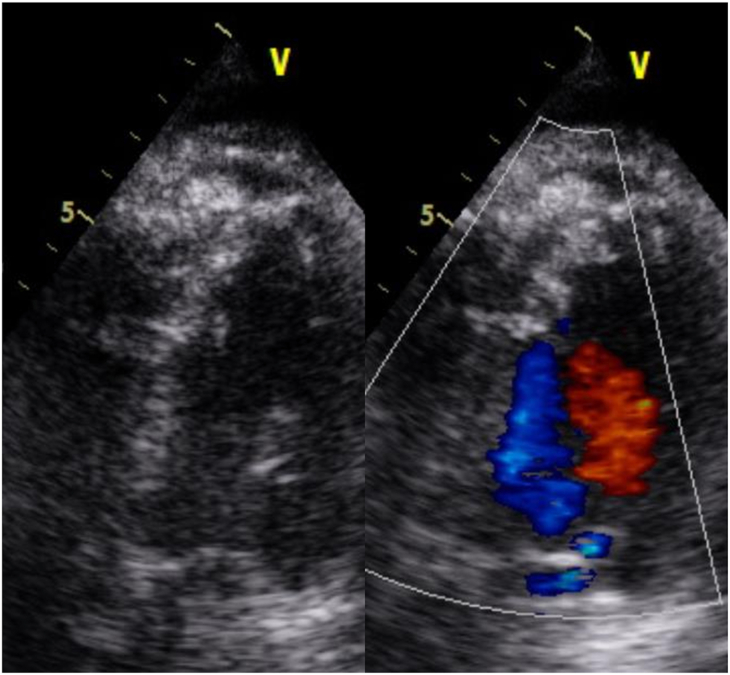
Postoperative transthoracic echocardiography showing the repaired dissected interventricular septum with no residual shunt. (5, 5 cm; V, ventral side).
